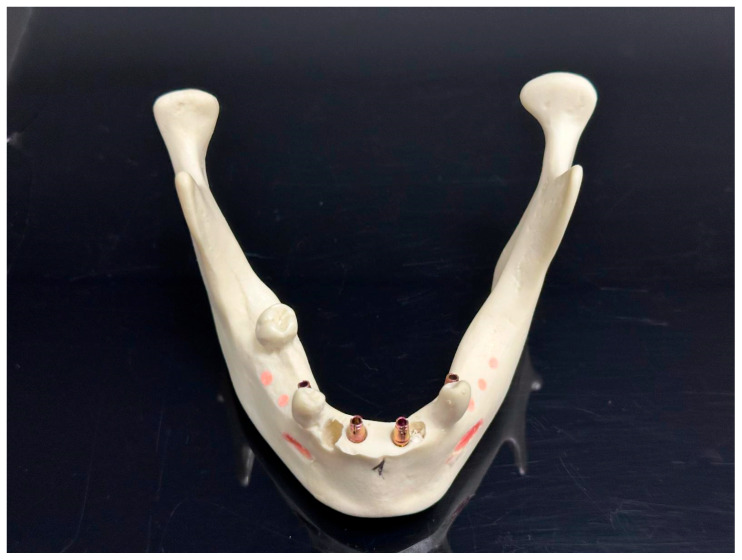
Trate root implants in place with the CRE carriers ready for CBCT exposition.
